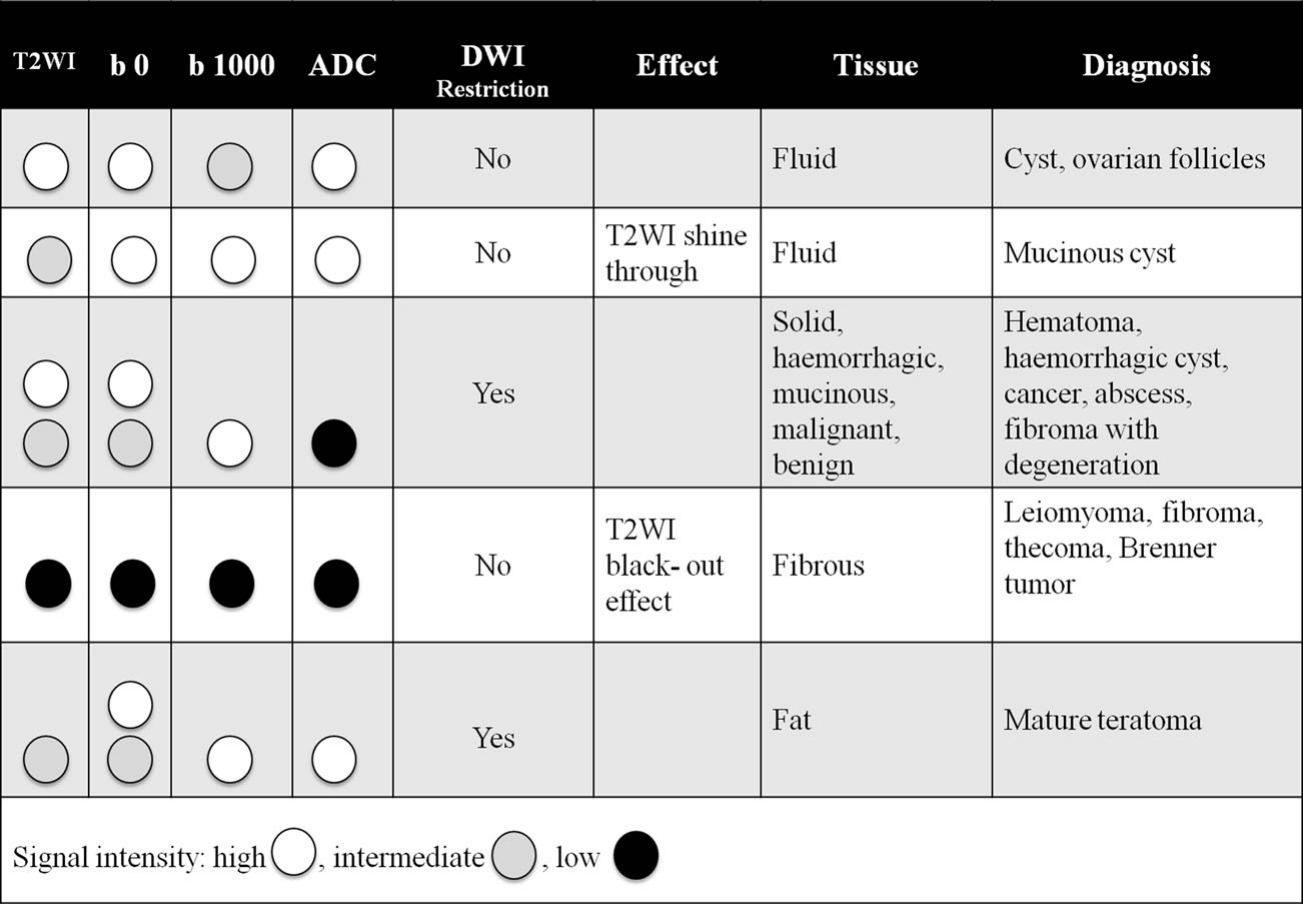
Characterization of adnexal masses by combining T2WI and DWI
